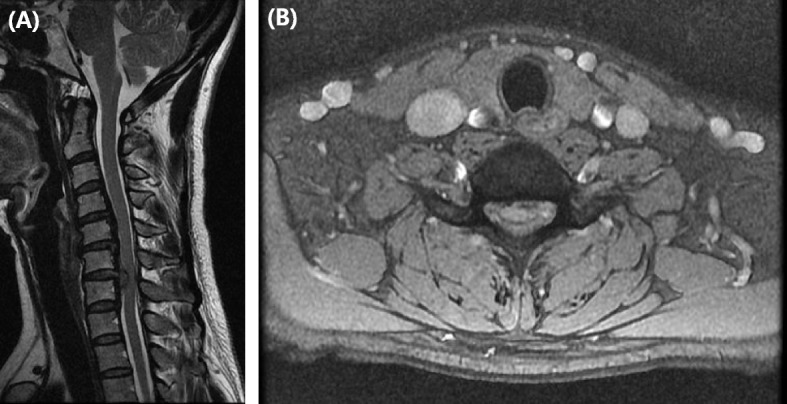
a Preoperative sagittal T2 weighted MR images showing large central disc extrusion with inferior migration, severe cord compression on C6–7 level. b Preoperative axial T2-weighted MRI showing severe C6–7 cord compression by a massive disc herniation
